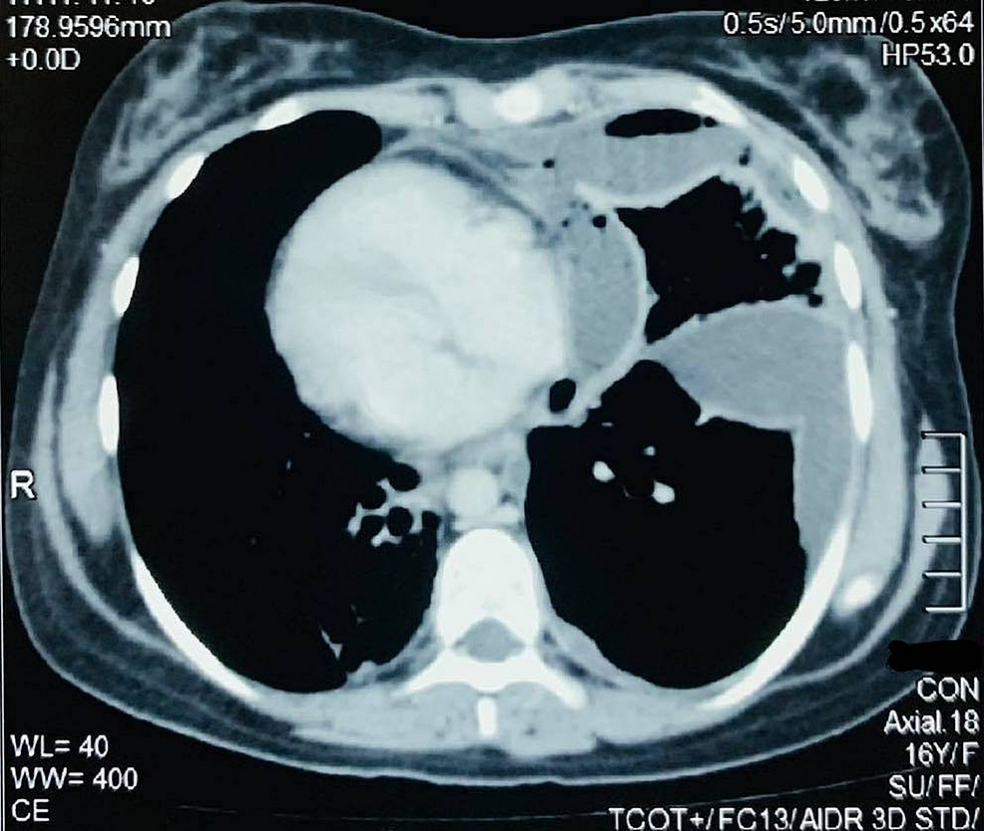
CT chest with IV contrast (axial view) showing dextrocardia and left lung empyema.
